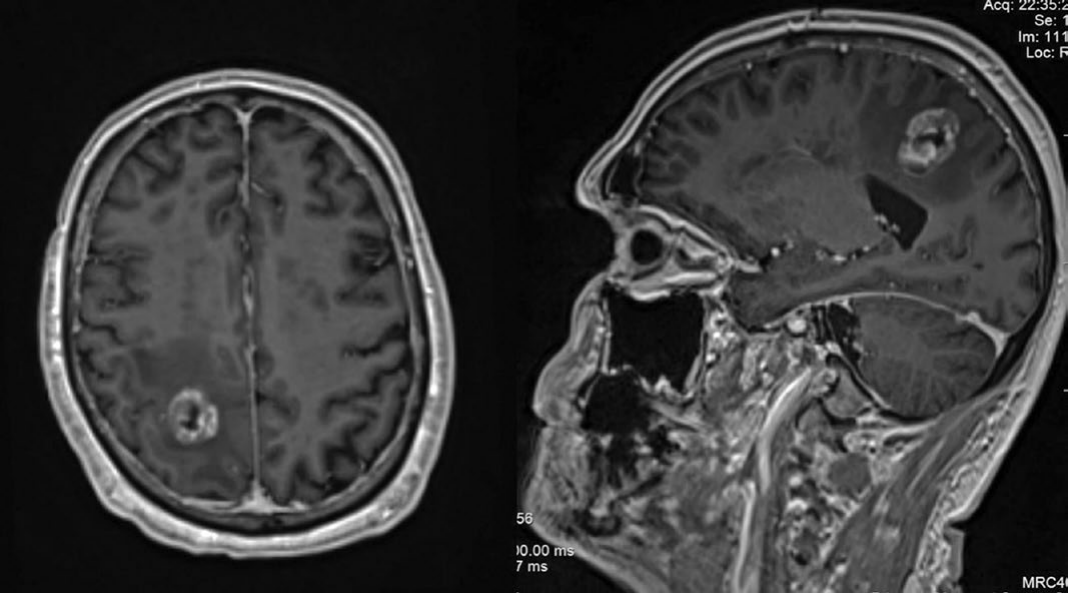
Intraparenchymal brain metastasis. Axial and sagittal T1-weighted MR images showing a heterogeneous enhancing metastasis in the right posterior semiovale, accompanied by surrounding vasogenic edema.
